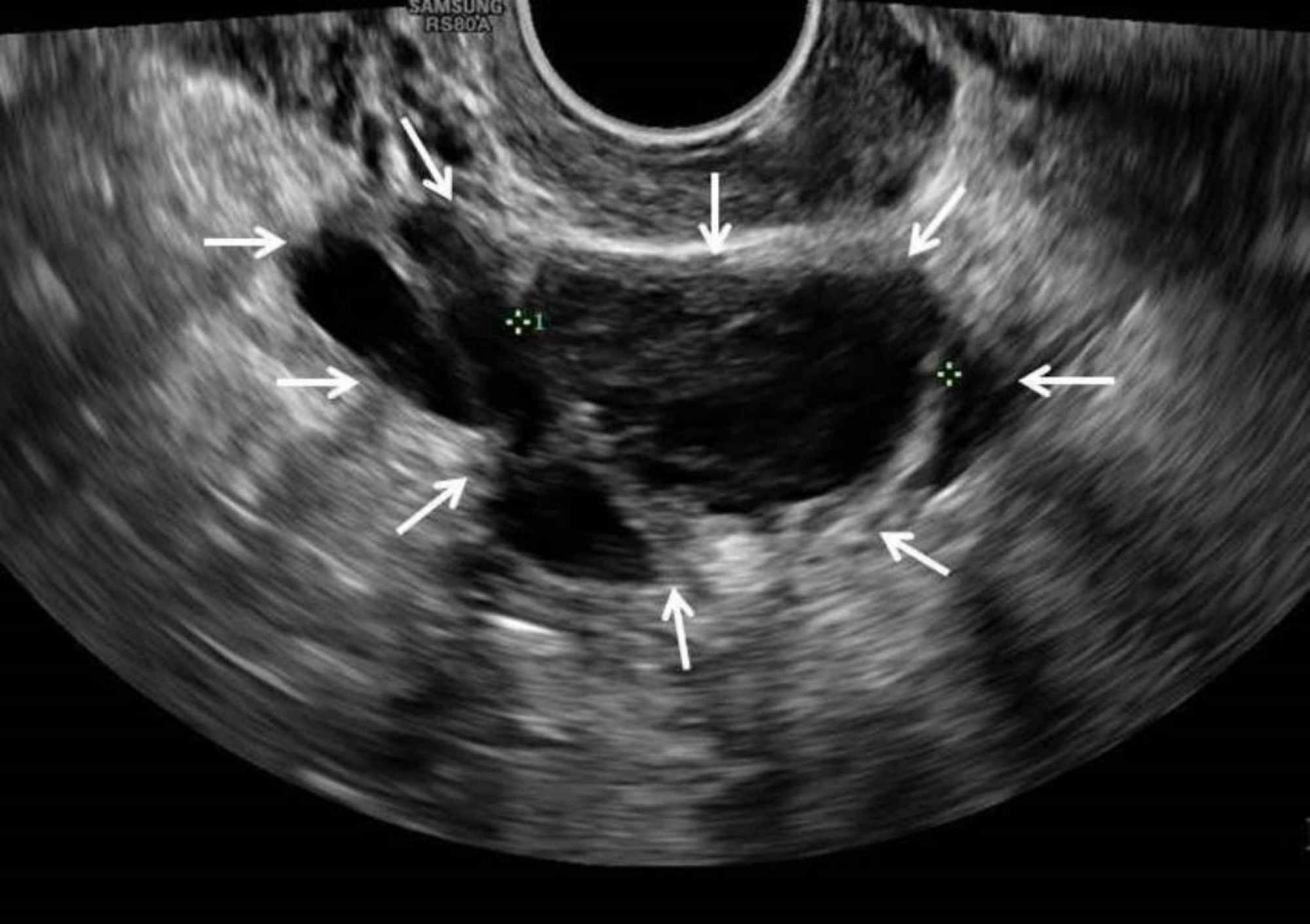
Ultrasound scan of the pelvis Demonstrating a small right ovarian cyst measuring 3.5 cm with internal echoes and stringy septa
